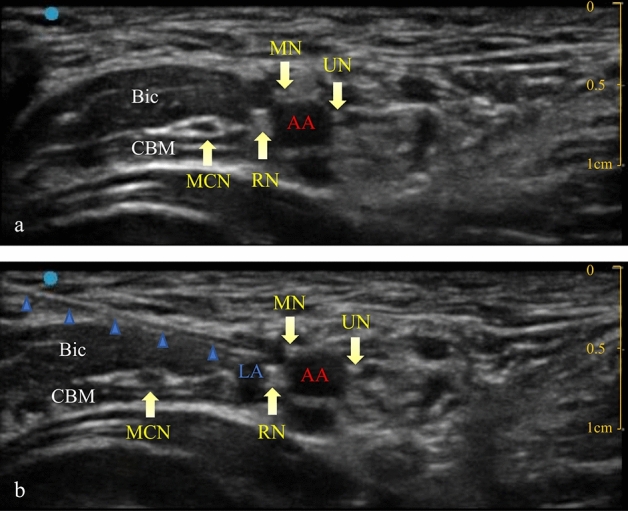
Ultrasound image preinjection (a) and ultrasound image postinjection (b). needle. AA: axillary artery; LA: local anaesthetics; MCN: musculocutaneous nerve; RN: radial nerve; MN: median nerve; UN: ulnar nerve; Bic: biceps brachii muscle; CBM: coracobrachialis muscle.
